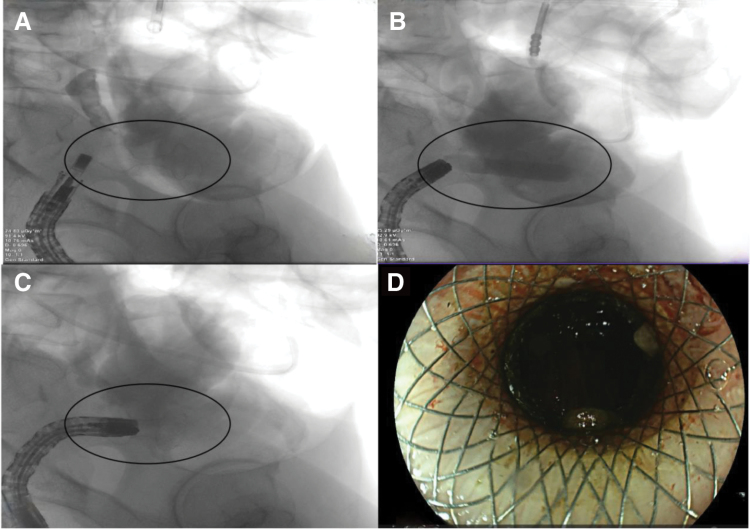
Step-by-step stent expansion with balloon (circled). A, X-ray fluoroscopy shows that the deployed stent is in good position, (B) use expansion balloon to expand the stent step by step, (C) X-ray fluoroscopy shows that the stent is well expanded, (D) endoscopy shows that the stent is well expanded.
